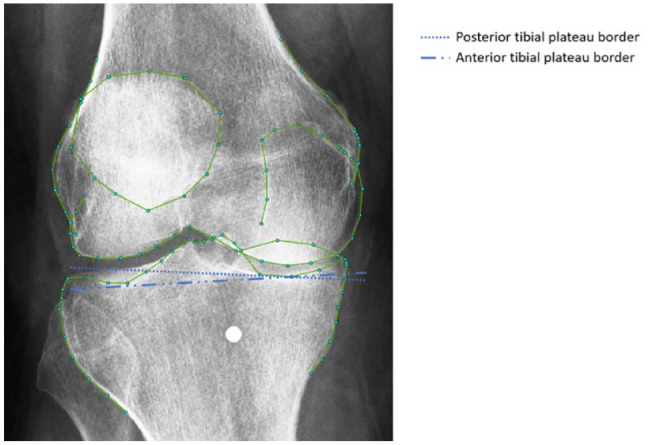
Skewed view of the tibial plateau on a knee radiograph obtained using the Buckland-Wright protocol. There are visible posterior and anterior lateral tibial borders, which are hard to distinguish. Selecting different borders affects the tibial joint line significantly.
